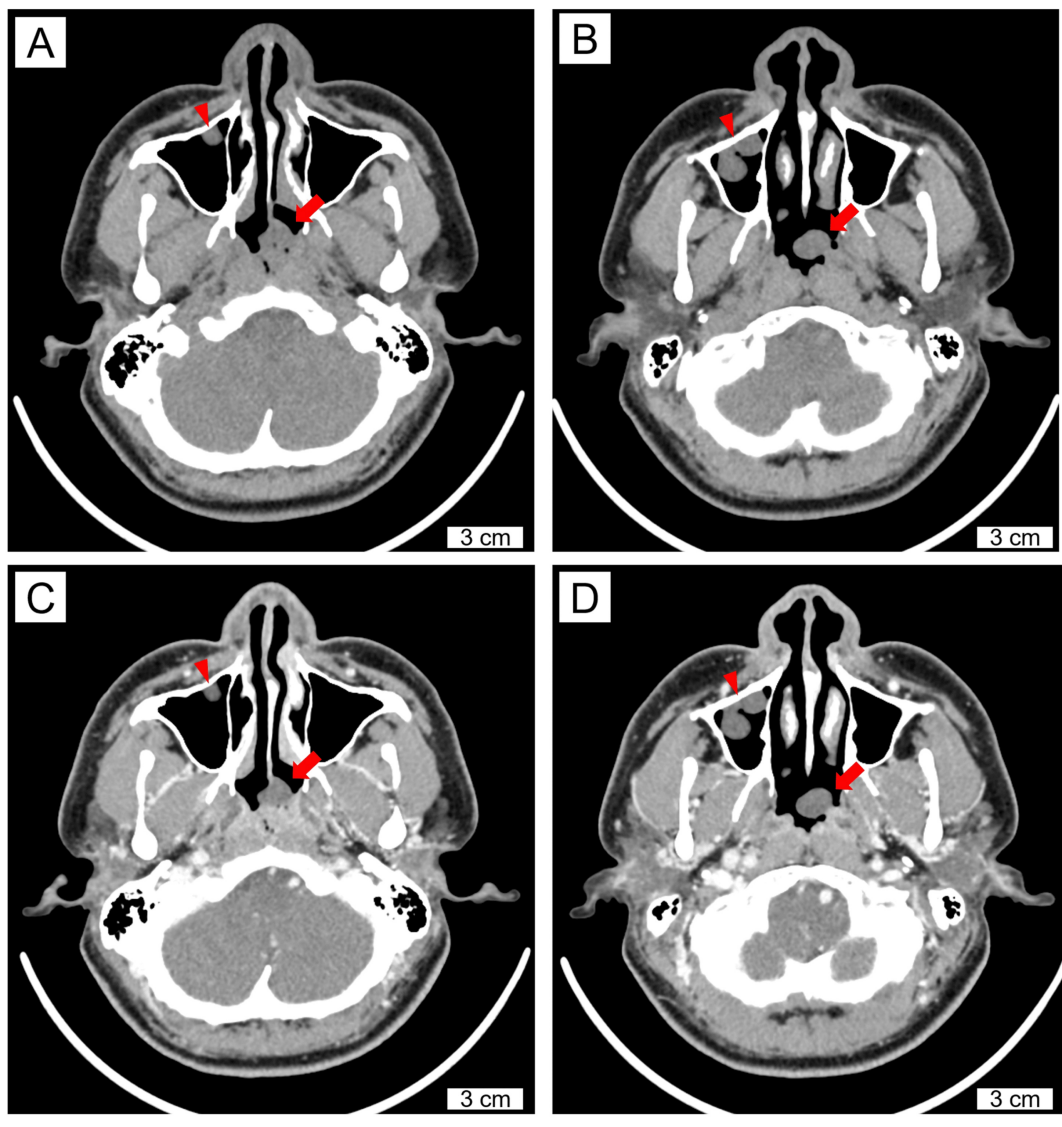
Computed tomographic images of the inflammatory nasal polyp with benign lymphangioendothelioma/acquired progressive lymphangioma. Computed tomography images (A, B: non-contrast-enhanced; C, D: contrast-enhanced; A corresponds to C; B corresponds to D) show a non-enhanced low-density lesion in the posterior nasal cavity (arrow) and two non-enhanced polypoid lesions in the right maxillary sinus (arrowhead). The nasal polyp attaches to the nasal septum in A and C. Scale bars are shown in the figures.
